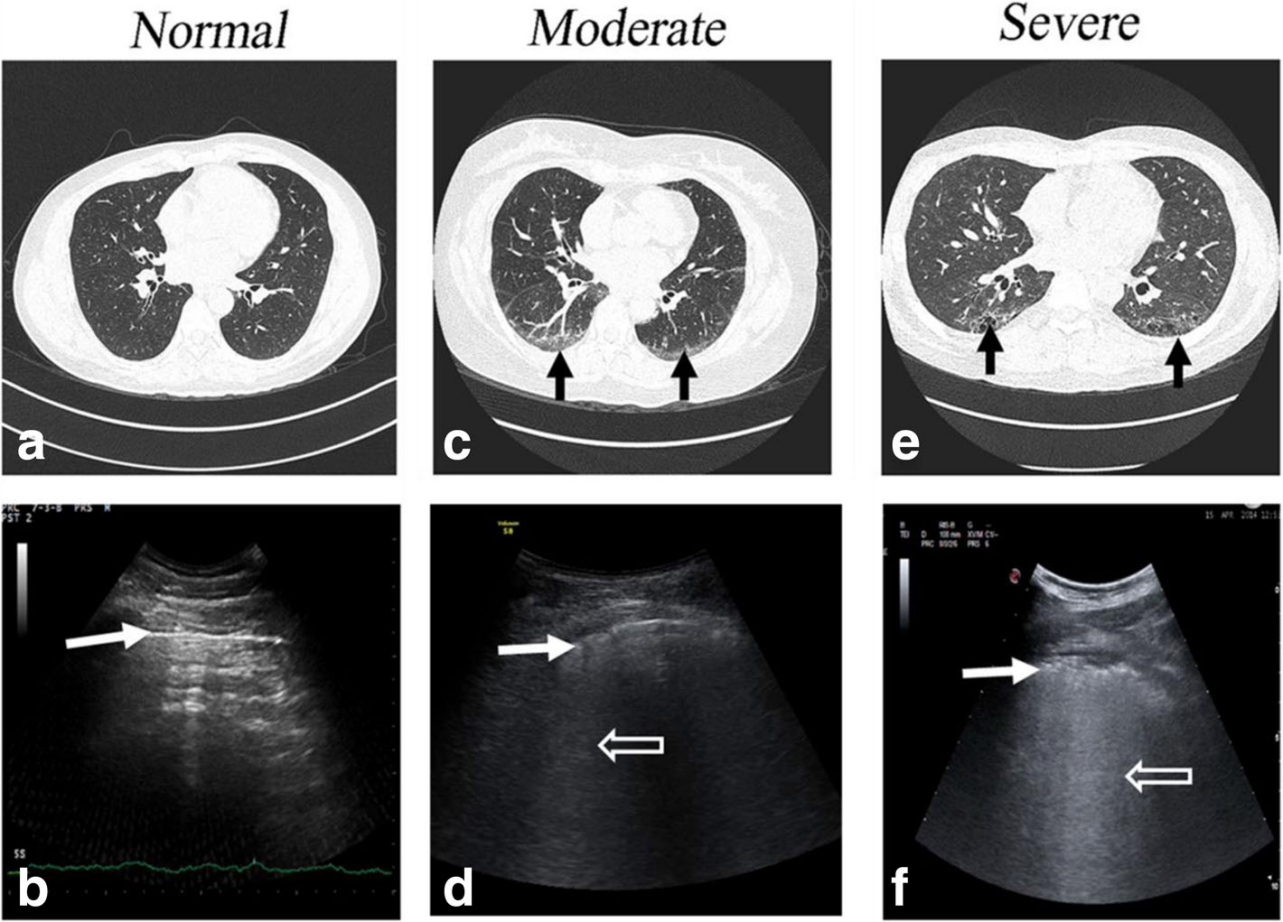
Different HRCT and LUS patterns. a HRCT: normal. b LUS: normal pleural line (white arrow). No B-lines visible. c HRCT: ground-glass opacity (black arrow). d LUS: blurred and irregular pleural line (white arrow) and multiple B-lines (white empty arrow). e HRCT: honey-combing (black arrow). f LUS: blurred and irregular pleural line (white arrow) and multiple B-lines (“white lung”, white empty arrow). HRCT high-resolution computed tomography, LUS lung ultrasound
